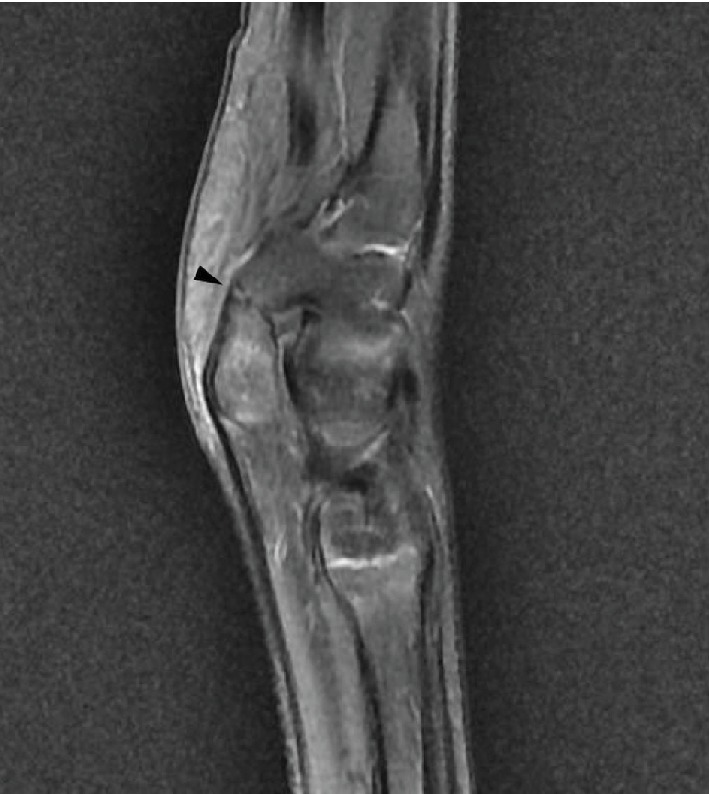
Preoperative MRI of the left wrist showing a synchondrosis coalition of the pisiform with the hook of the hamate (arrowhead).
